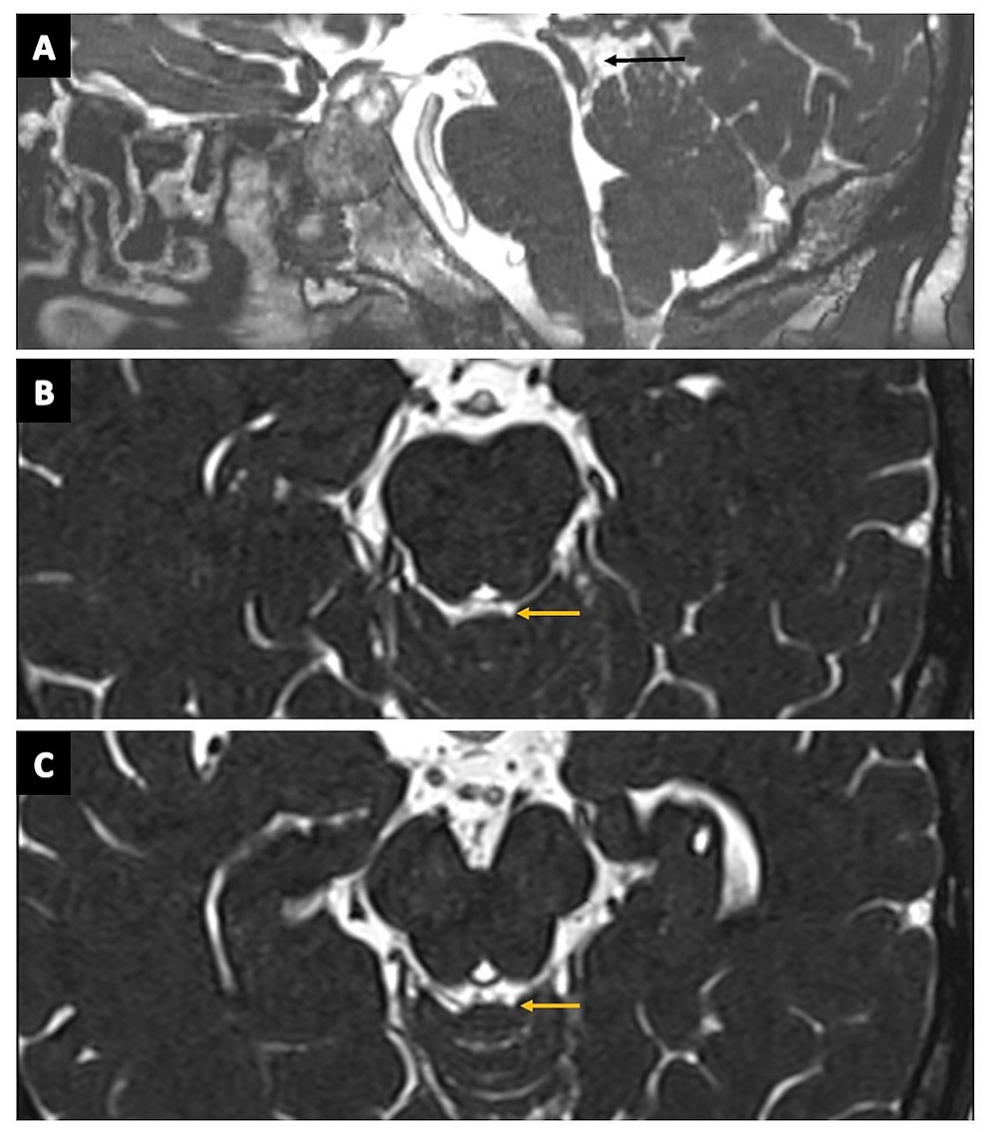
The cisterna quadrigeminalis (quadrigeminal cistern). (A) The quadrigeminal cistern (arrow) in the sagittal section of the three-dimensional (3D) constructive interference in steady state (CISS) MRI sequence. (B) and (C) show the quadrigeminal cistern (arrows) as seen on the axial planes of 3D-CISS MRI sequence.
